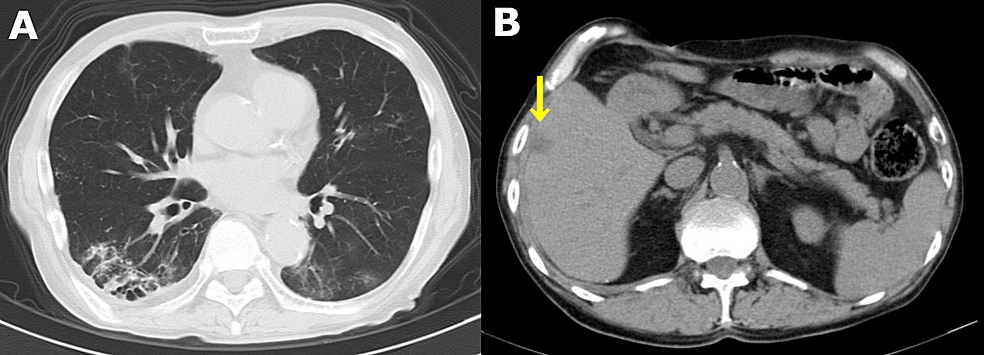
CT findings in the lung and liver A: Mottled gland-glass opacity with an emphysematous change in the bilateral lower lobes. B: SOL in the S5 of the liver (yellow arrow) CT: computed tomography; SOL: space-occupying lesion
